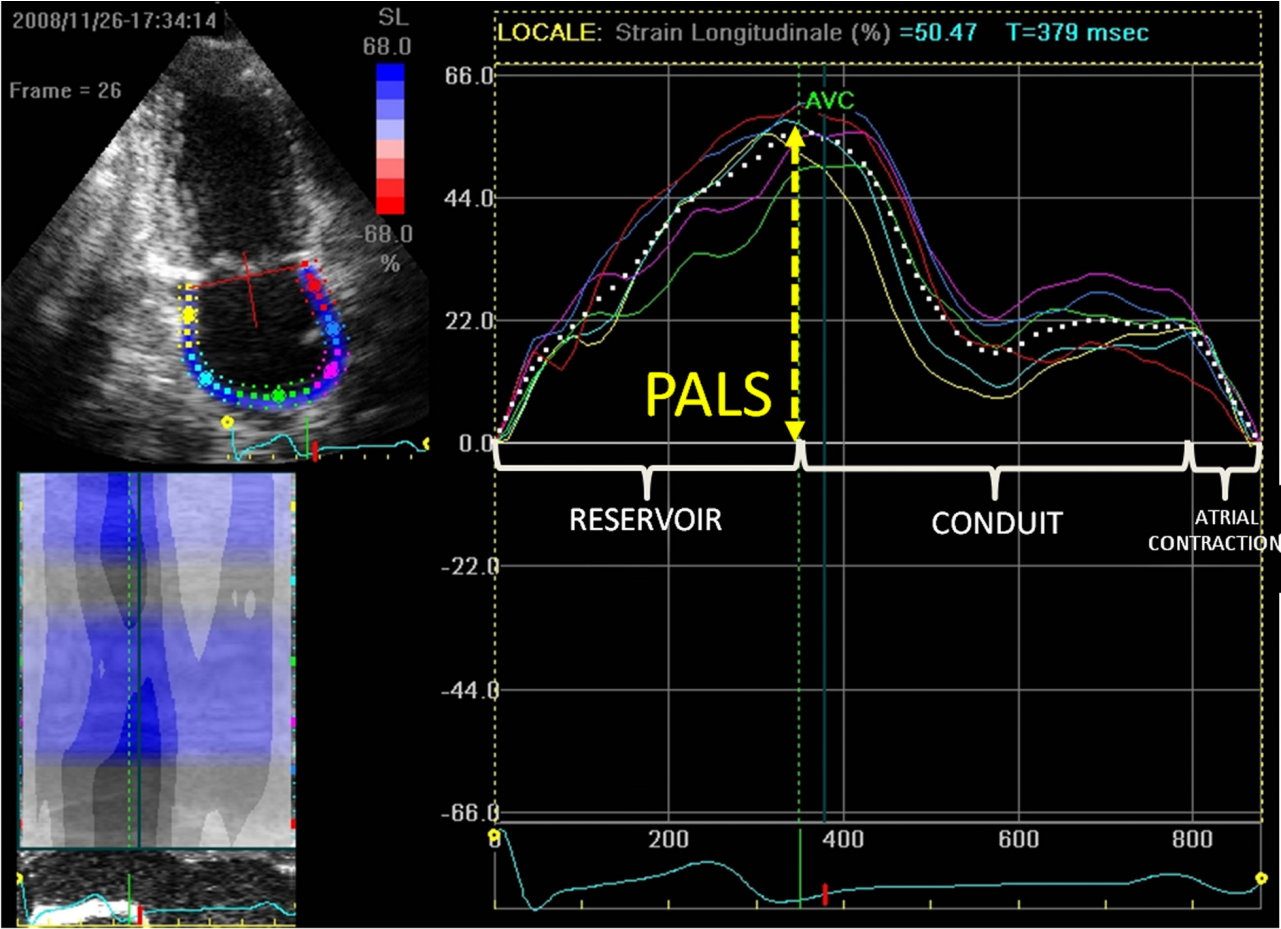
Measurement of peak atrial longitudinal strain (PALS) from an apical two-chamber view. The dashed curve represents the average atrial longitudinal strain along the cardiac cycle. (PALS, peak atrial longitudinal strain; AVC, aortic valve closure).
